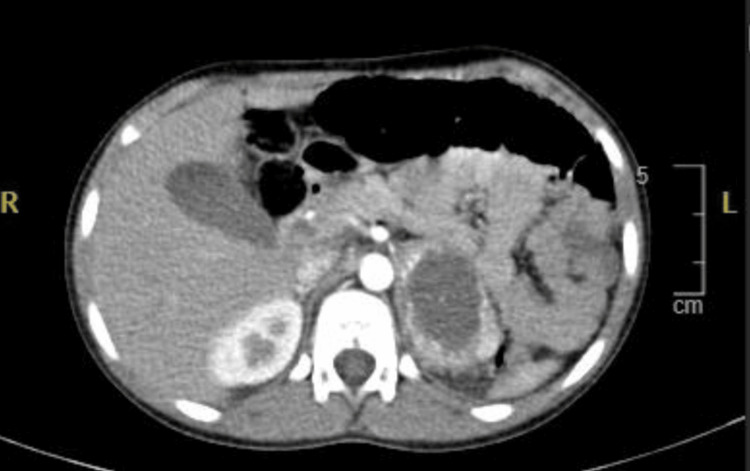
Axial contrast-enhanced abdominal CT scan demonstrating a large left adrenal pheochromocytoma with central necrosis CT: computed tomography
